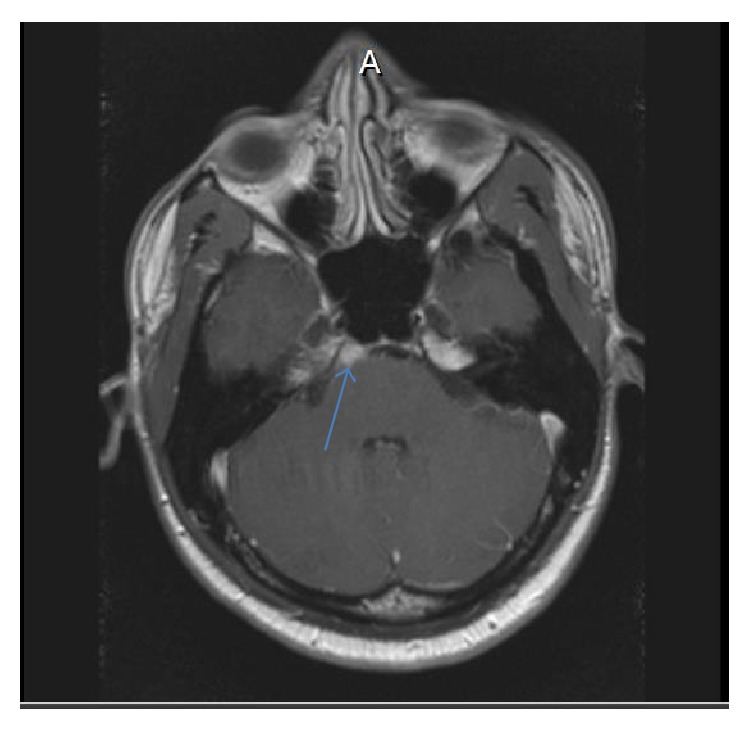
MRI of brain T1 axial + gadolinium showing small tuberculoma in the right prepontine cistern before anti-TB treatment.
